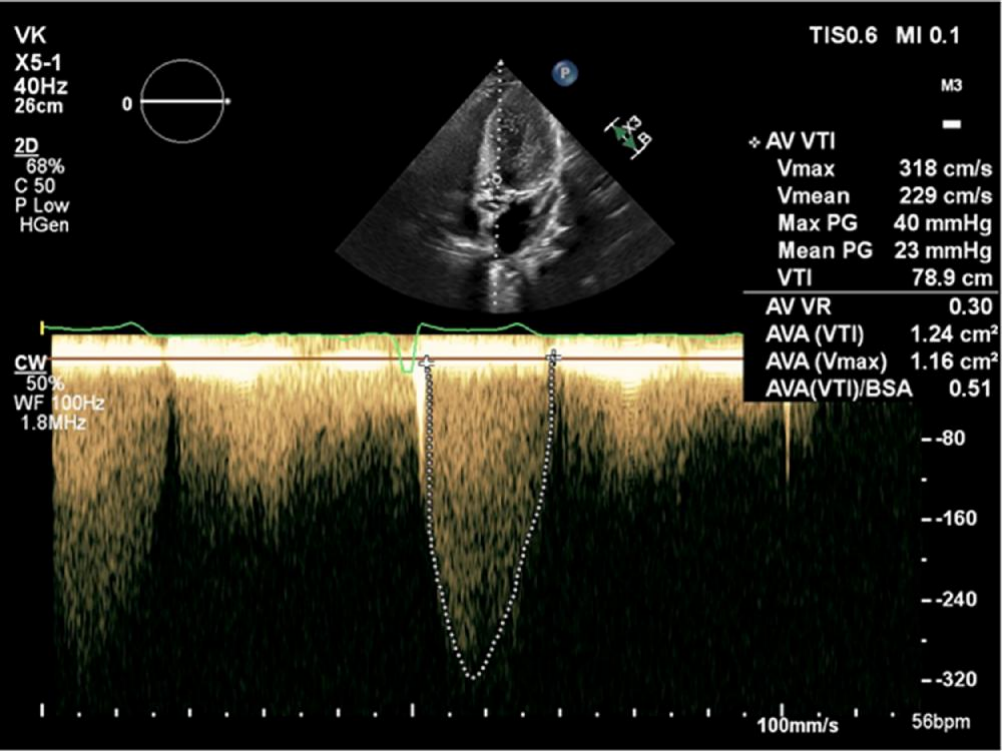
Transthoracic echocardiogram apical five-chamber view showing poor continuous wave waveform through the aortic valve.
